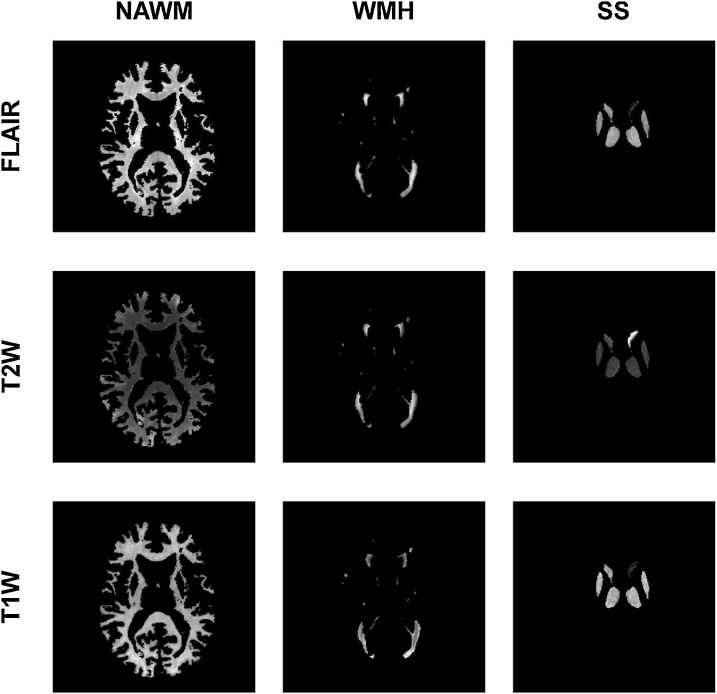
Set of images obtained in each patient. In this representative case, T1-weighted (T1W), T2-weighted (T2W) and fluid-attenuated inversion recovery (FLAIR) brain images of normal appearing white matter (NAWM), white matter hyperintensities (WMH) and subcortical structures (SS) of a lacunar stroke patient are presented.
